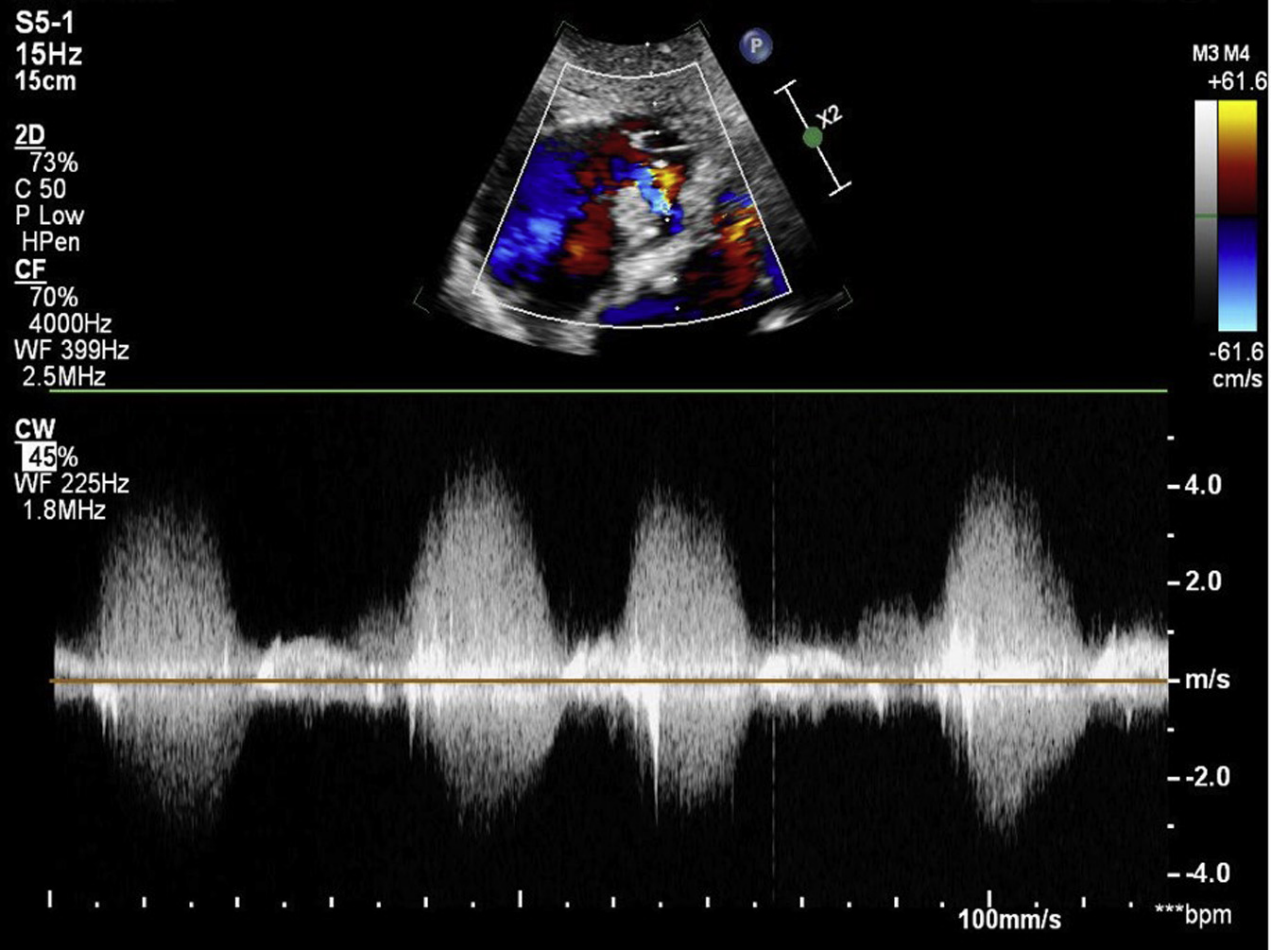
Continuous-wave Doppler through Gerbode defect. Peak velocity is 4.7 m/sec.
